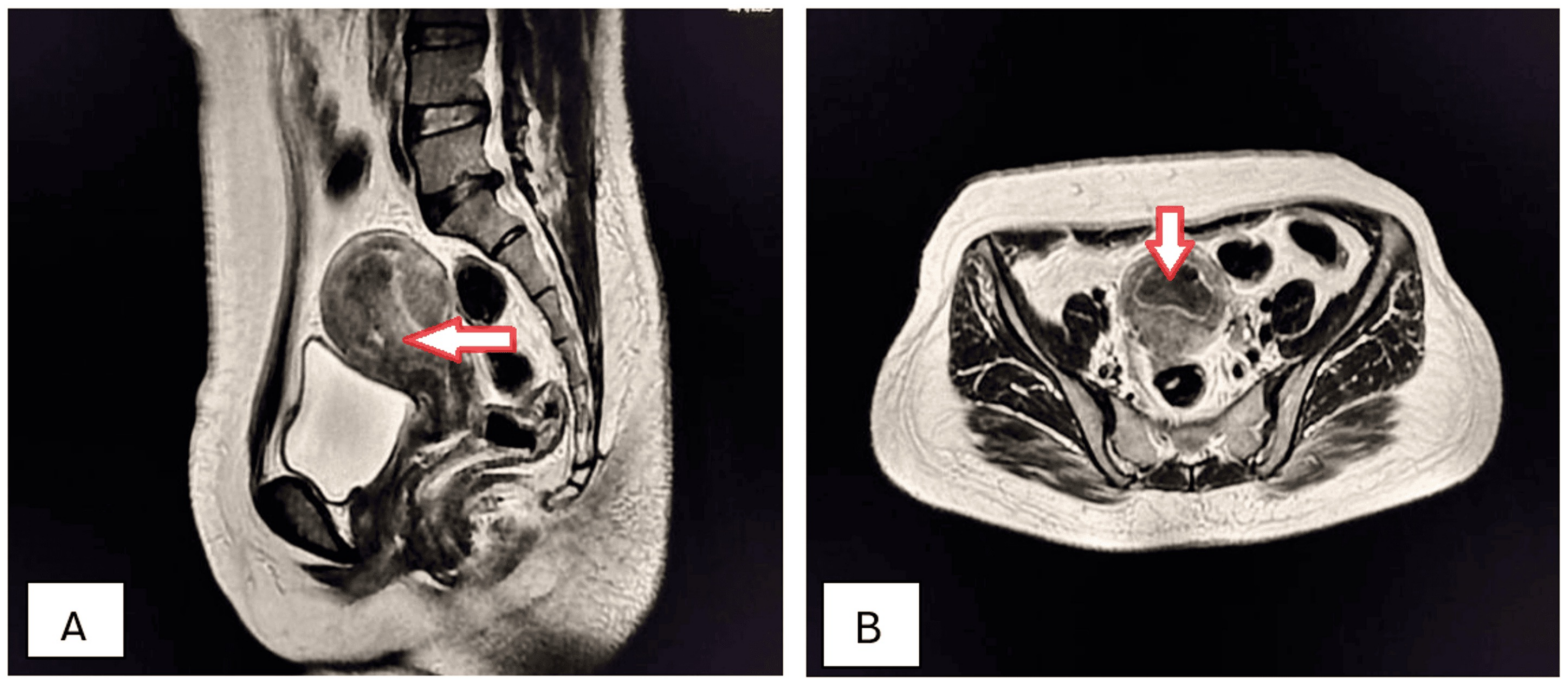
Retained products of conception (arrows). Sagittal (A) and axial (B) T2W MRI imaging shows inhomogeneous endometrial collection with peripheral, hyperintense endometrial lining, consistent with retained products of conception (an incomplete abortion). T2W: T2-weighted
